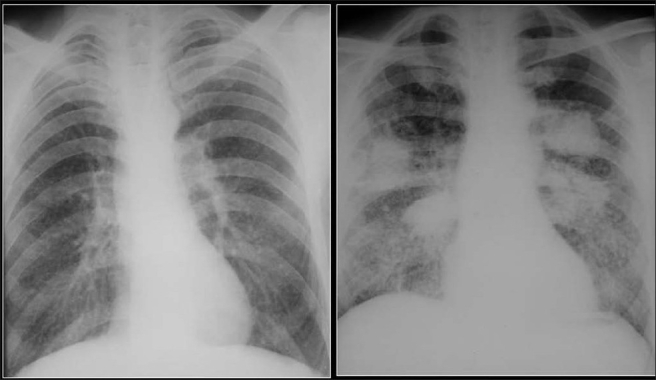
Two chest radiographs from the same Iron ore worker 10 years apart shows fine high-density nodules (left) progressing to PMF 10 years later
